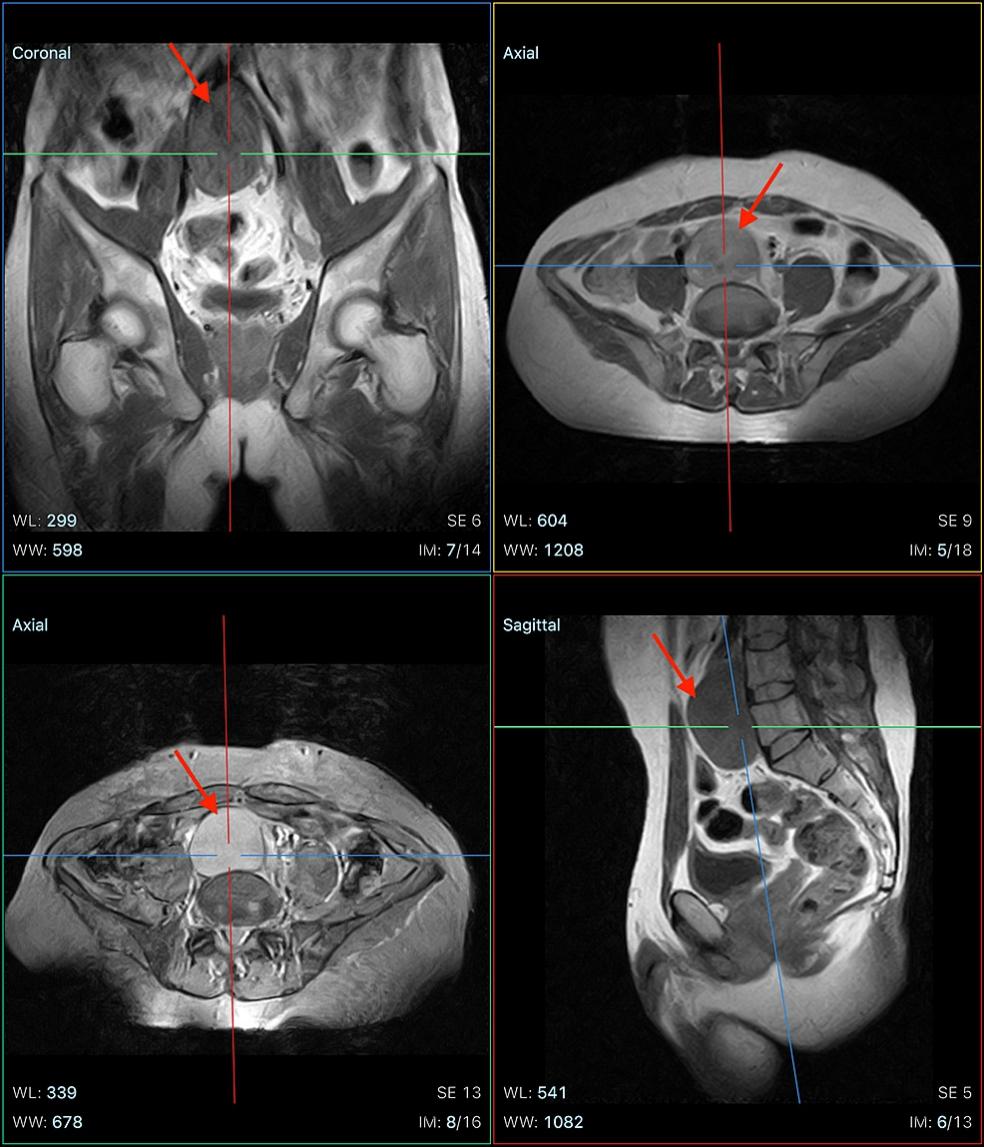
MRI pelvis showing a retroperitoneal mass in the prevertebral space, along L4 and L5 vertebrae, with dimensions of 7.5 cm × 6 cm × 5.5 cm (TV × AP × CC) in coronal (top left), axial (top right, bottom left), and sagittal (bottom right) views. MRI: magnetic resonance imaging; TV: transverse; AP: anteroposterior; CC: coronal
